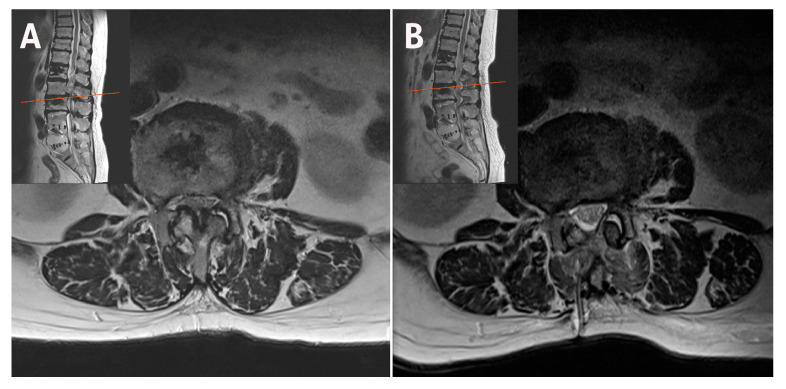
(A) MRI demonstrating vertically oriented facets at the L2–L3 level associated with ASS. (B) Postoperative MRI showing decompressed L2–L3 recesses in a patient with ASS and preserved orientation of both facets. MRI, magnetic resonance imaging; ASS, adjacent segment stenosis.
